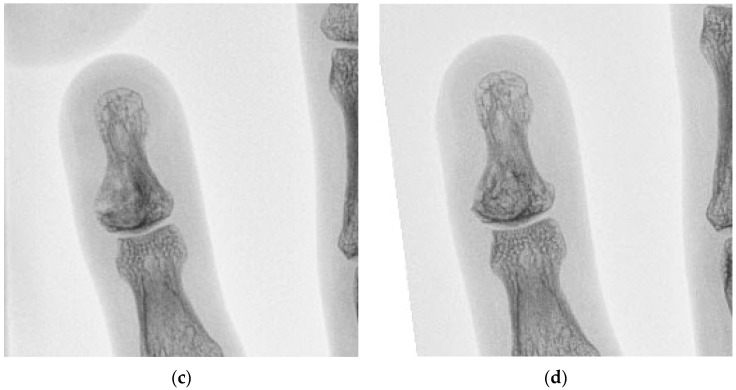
Posteroanterior (PA) radiographs of left little finger distal phalanx (*Case 5*): (a) preoperatively, showing an enchondroma at the base of P3; (b) immediately postoperatively, following excision and curettage, with the contained defect filled with GeneX; (c) at 6 months follow-up, the GeneX shows partial resorption and there is some evidence of bone healing; and (d) at 12 months follow-up, the GeneX has been completely resorbed and the affected cortex has largely remodelled.
